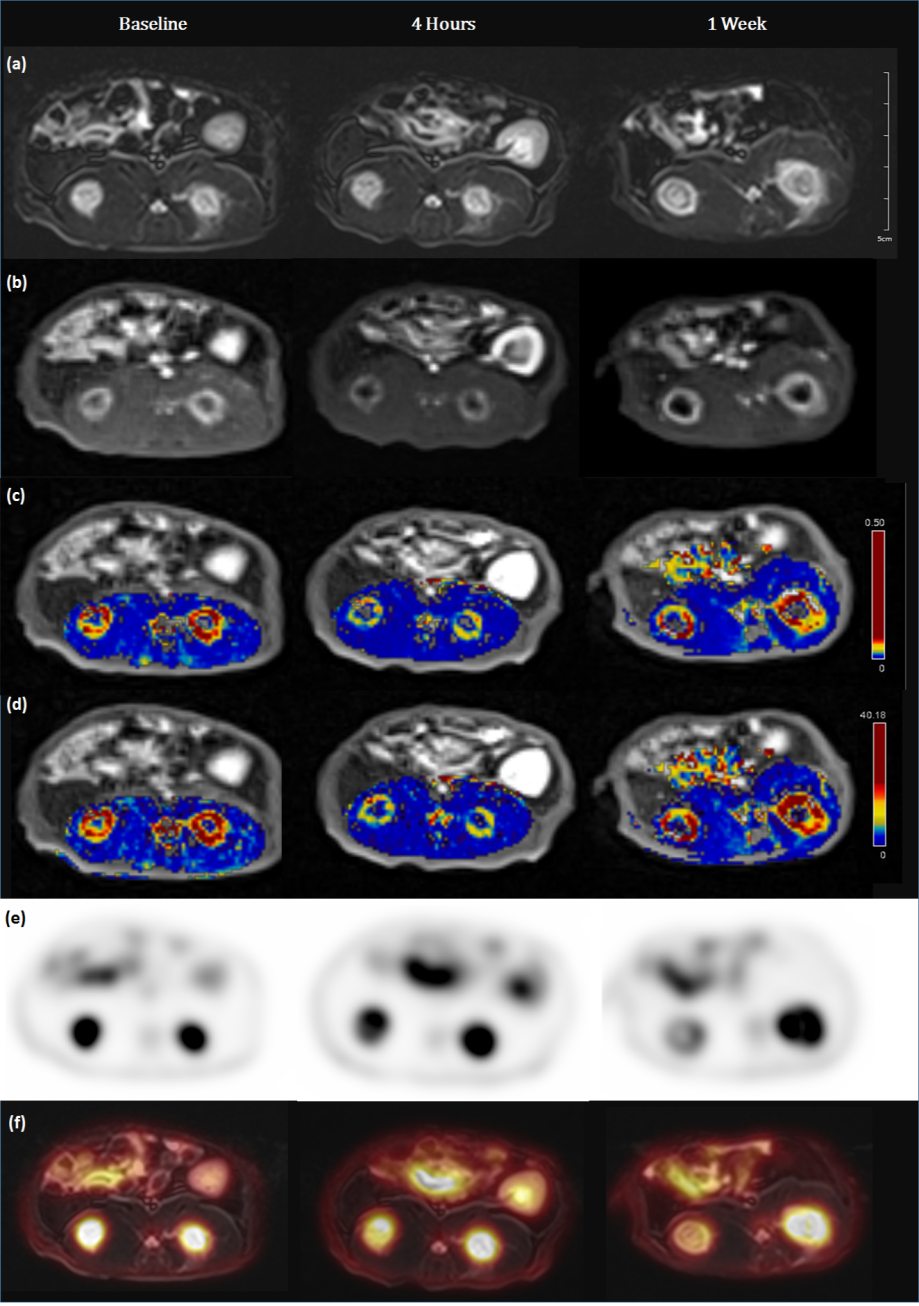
Serial changes of PET/MRI parameters before and after the treatment with CKD-516. (a) Axial T2-weighted images demonstrated target tumors with high signal intensity in bilateral paravertebral muscles. (b) DCE-MRI revealed peripheral enhancement of tumors suggesting central necrosis. (c) K^trans^ map and (d) iAUC map demonstrated a reduction of values at 4 hours and partial recovery at 1 week follow-up. (e) PET images and (f) fusion images showed no significant differences of SUVmax or SUVmean.
